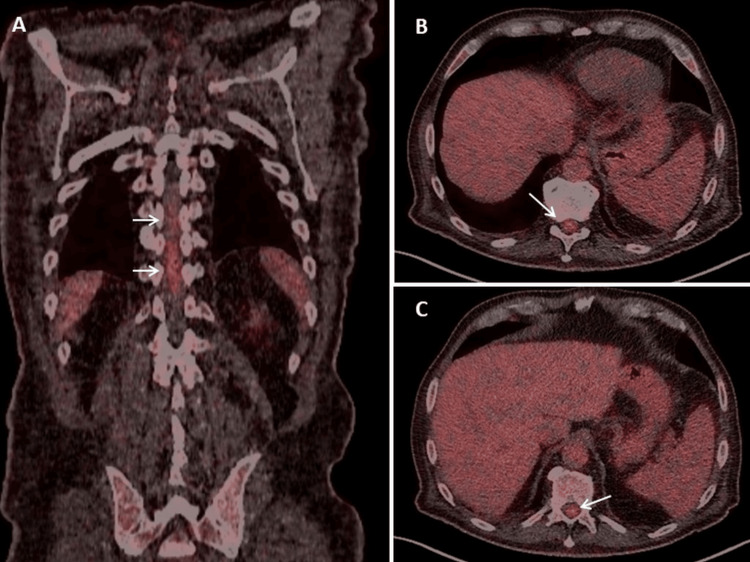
FDG-PET coronary (panel A) and axial planes (panels B and C) showing a linear uptake at the level of T10/T11 in the spinal cord (SUVmax 5; Deauville 5) (arrows), which can be interpreted as either infiltration of the lymphoma or carcinosis FDG-PET: fluorodeoxyglucose positron emission tomography
